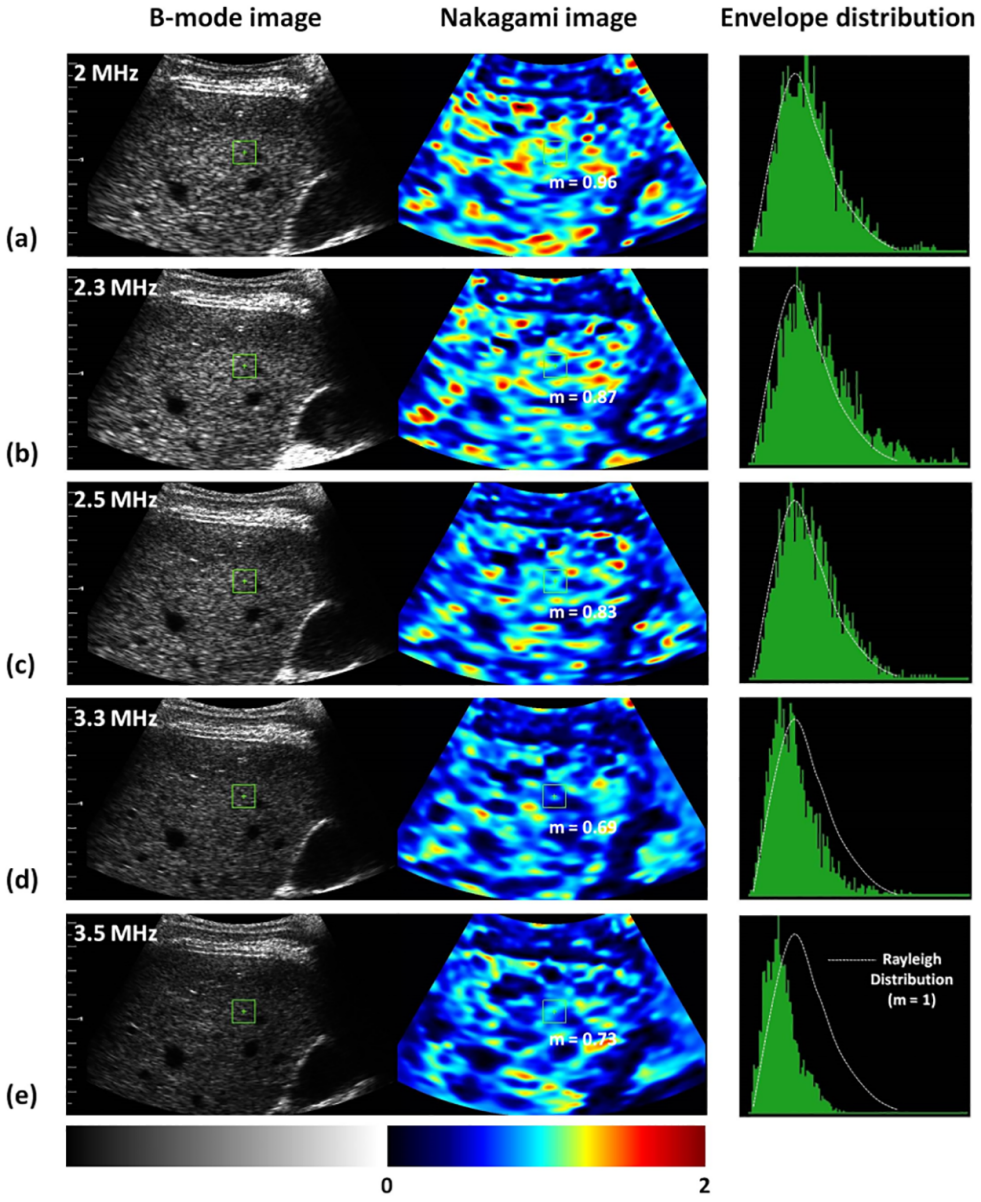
Typical B-scan, Nakagami images, and the envelope distributions of liver tissues obtained at ultrasound central frequencies ranging from 2 to 3.5 MHz. The envelope distributions were expressed using the histograms (plotted with 100 green bins). The white dotted lines mean the Rayleigh distribution. The shading of the Nakagami images gradually varied toward blue with the increasing ultrasound central frequency, corresponding to changes in the echo amplitude distribution of the signals acquired in the ROI to the pre-Rayleigh distribution.
